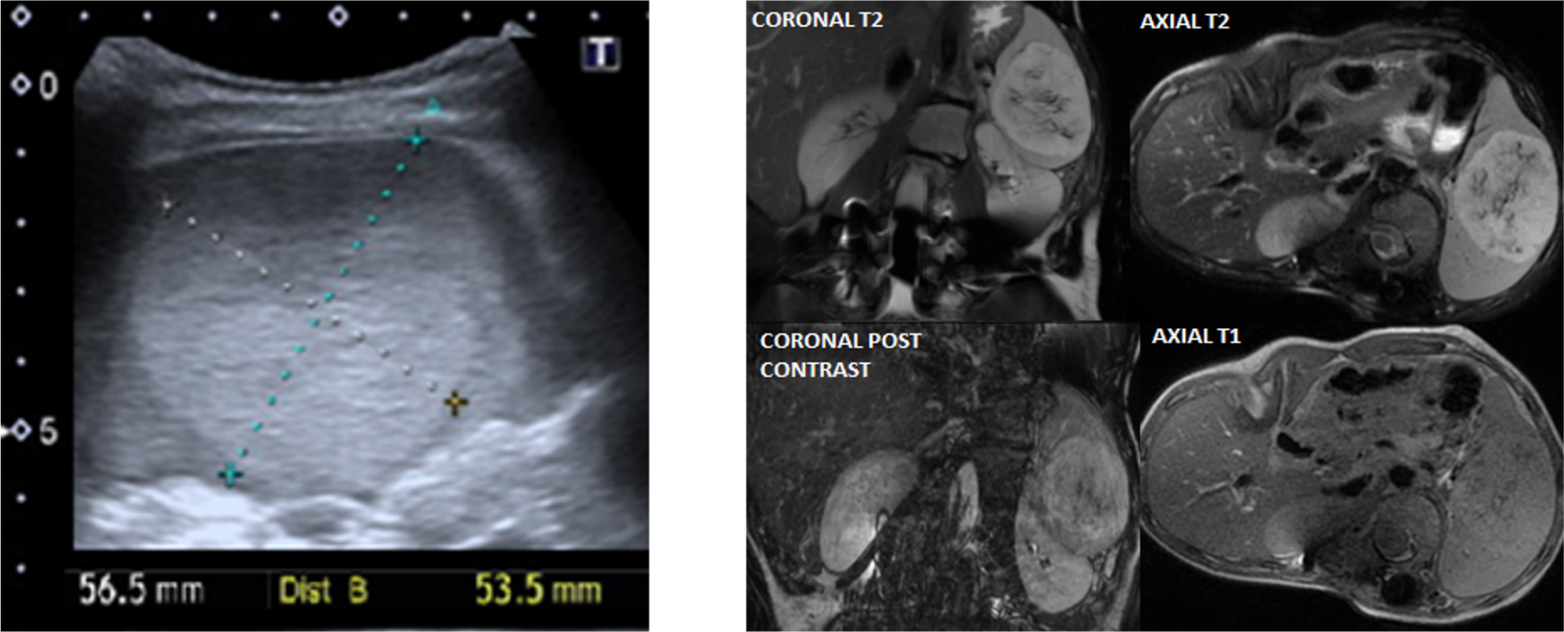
Splenic hamartoma clinical imaging. Left panel: Ultrasound of a patient with well-defined splenic solid mass, 5.7 × 5.4 centimeters, isoechoic, with calcifications dotted inside. Right panel: MRI imaging of the same patient that shows stable mass of 7.2 × 5.9 × 6.9 cm, hyperintense in T2, isointense in T1, without fat, with enhancement in small hemorrhagic areas.
